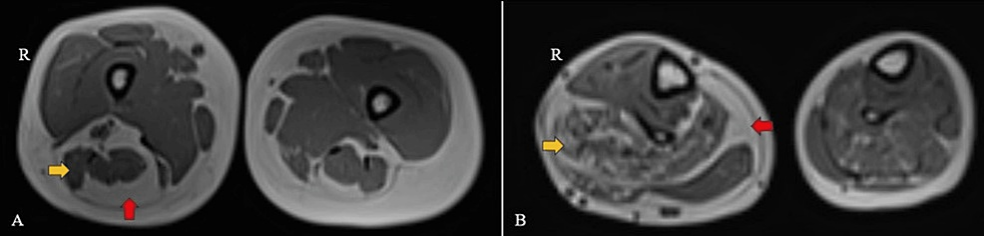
An MRI of the bilateral lower limb (axial, T1WI images) shows muscle atrophy (yellow arrows) accompanied by hypertrophy of the intermuscular and subcutaneous fat planes (red arrows) in the right thigh (A) and leg (B). T1WI: T1-weighted imaging
